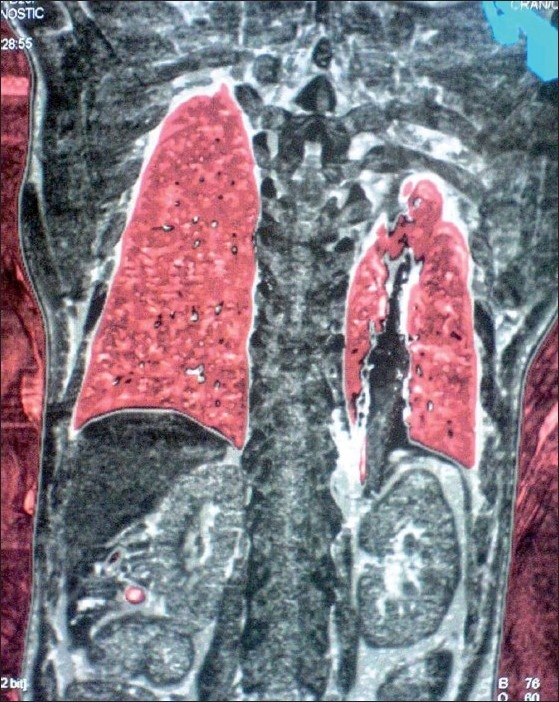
Three-dimensional virtual CT scan image showing the track followed by the arrowhead that now lies with its tip just in touch with upper and middle pole of left kidney. It has not caused any pneumo/hemothorax
